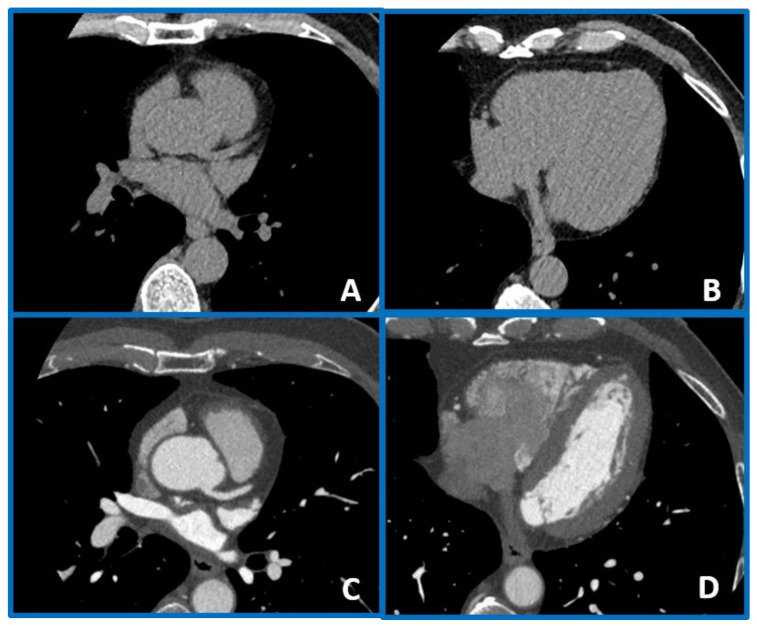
A case example of a 50-year-old man with arterial hypertension and noncardiac chest pain in which a coronary artery calcium score (CACS) value of 0 (panels (A,B)) was associated with no coronary plaque at coronary computed tomography angiography (CCTA) (panels (C,D)). In this case, CCTA evaluation did not add any information on top of CACS.
